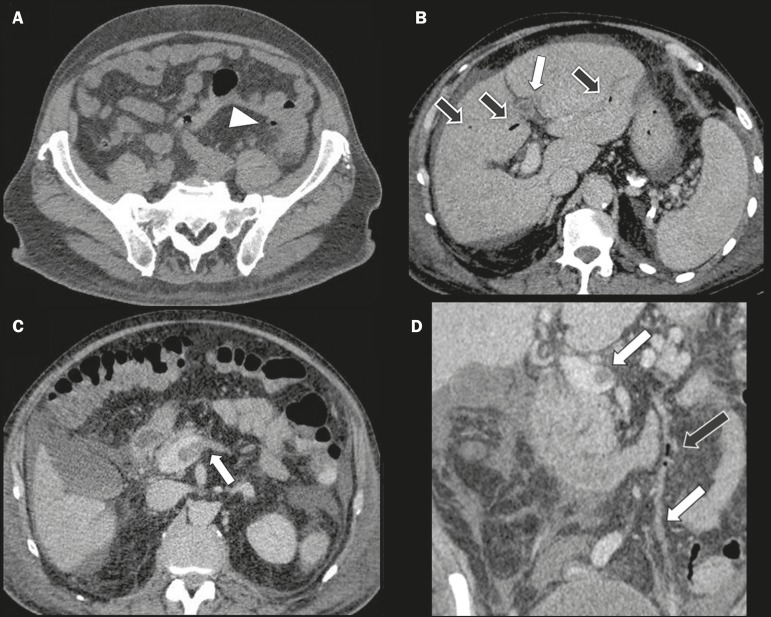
A: Non-contrast-enhanced abdominal CT of the patient at admission, showing
